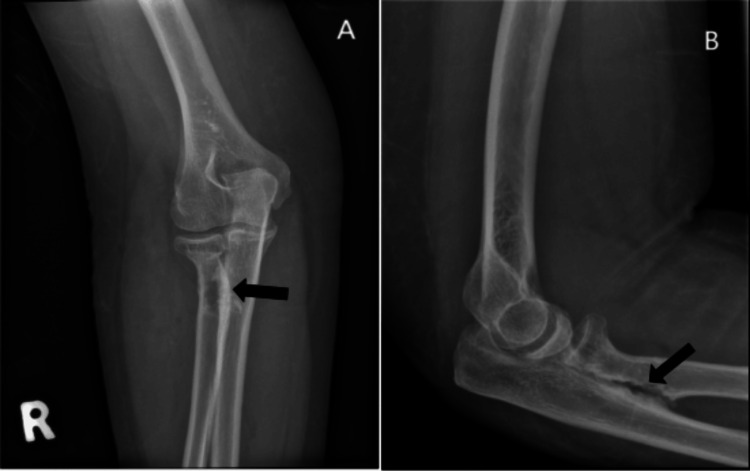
Postoperative radiograph AP view of the right elbow (A) and lateral view of the right elbow (B), with the arrow showing no interval calcification or remnant of ossification AP: anteroposterior
